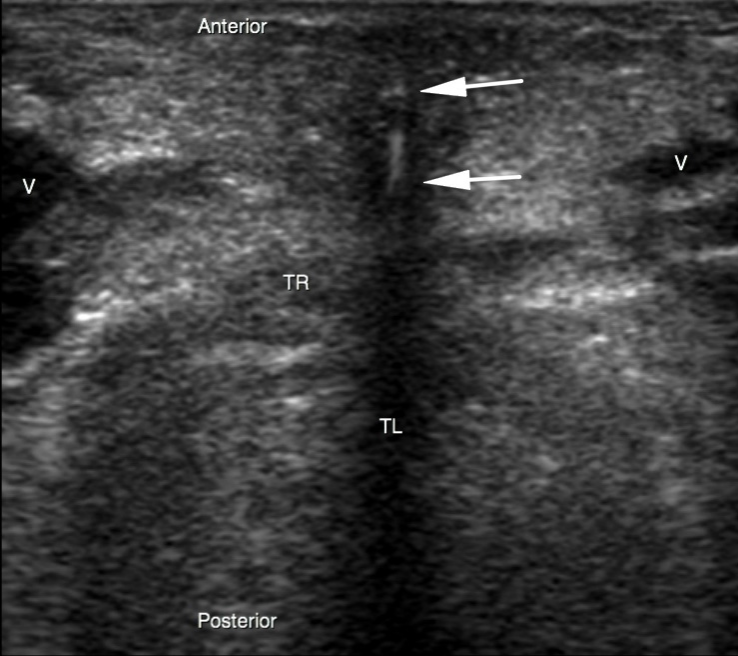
Real-time ultrasound guidance using an out-of-plane approach. The dilator is determined by a hyperechoic signal centered by a distinct acoustic shadow (arrows). TL, trachea lumen; TR, tracheal ring; V, vessel.
